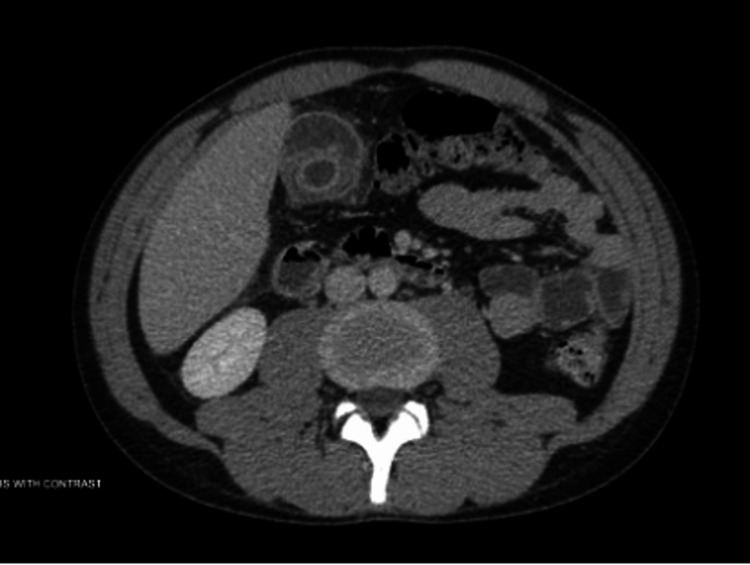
CT of the A/P with persistent heterogenous wall thickening of the gallbladder with intact outer wall and mild increased intraperitoneal fluid Gallbladder distention and adjacent inflammation suspicious for cholecystitis A/P: abdomen and pelvis
